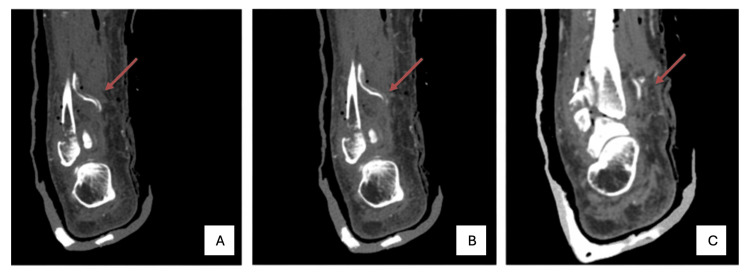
CT scan coronal view Coronal plane CT angiography showing the course of the dominant PTA from lateral (A) to medial (C) at the level of the ankle. PTA, posterior tibial artery
